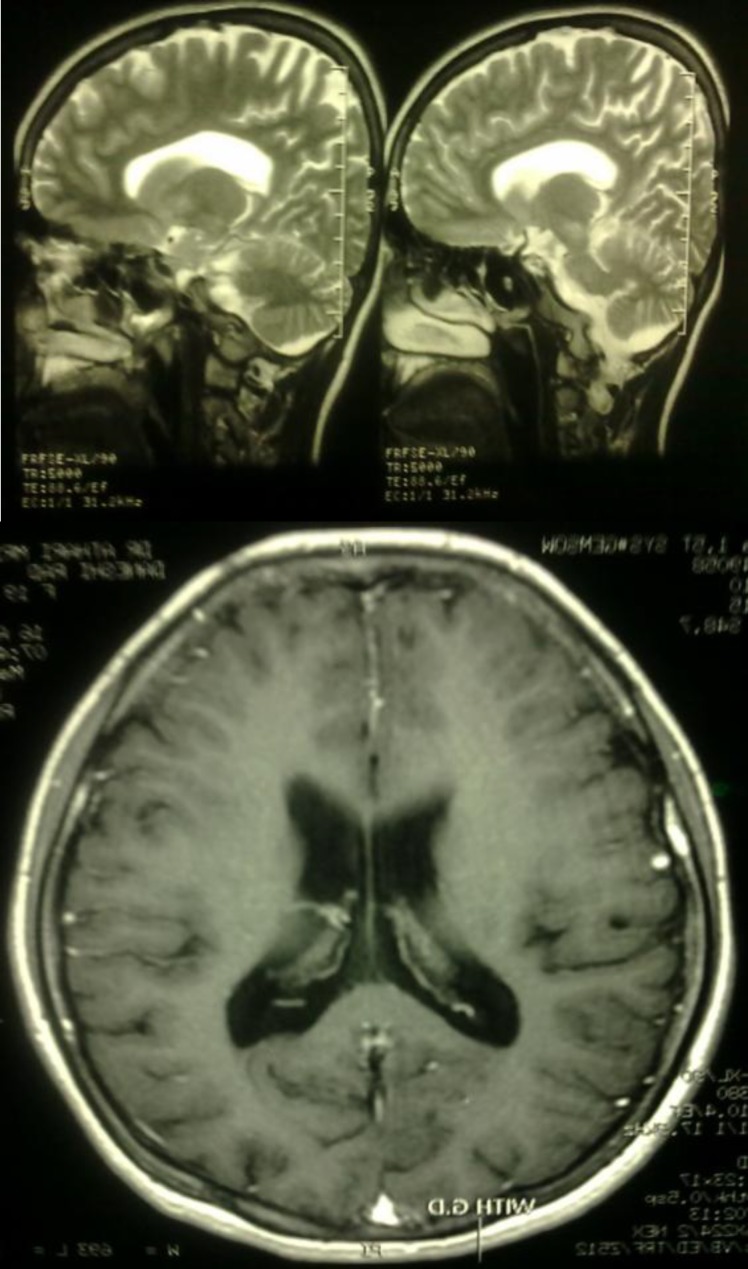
Brain MRI. signal abnormality in the bilateral basal ganglia, brain stem and superior cerebellar peduncles without post-contrast enhancement
